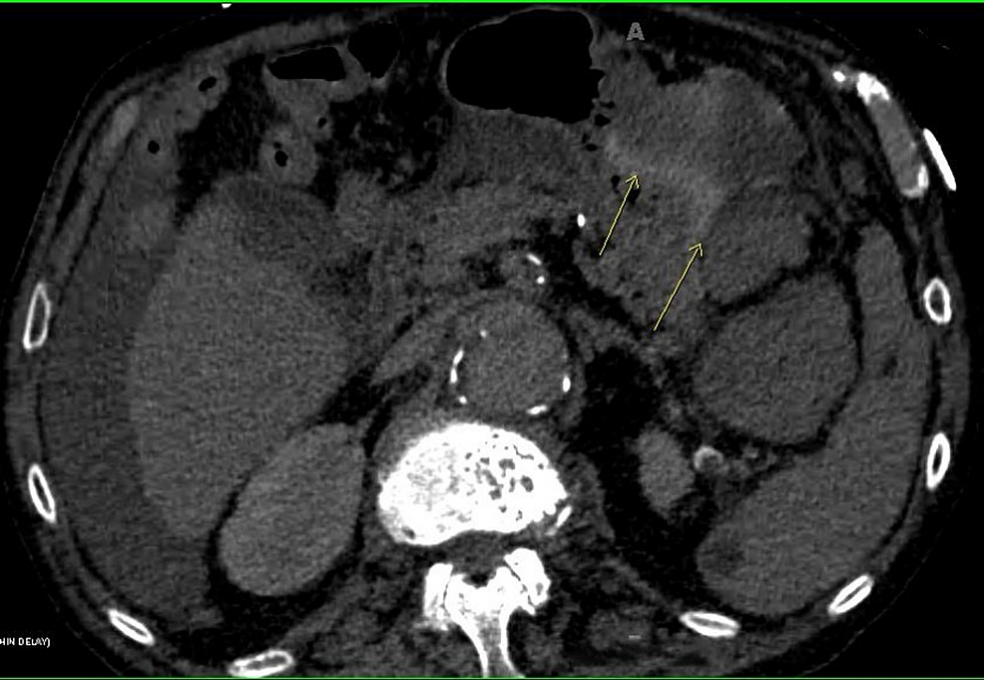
Computed tomography (CT) of the abdomen and pelvis revealed a hematoma in the sub-diaphragm, gastrosplenic ligament, hyper densities around the liver, and bilateral paracolic gutters extending into the pelvis (arrows).
